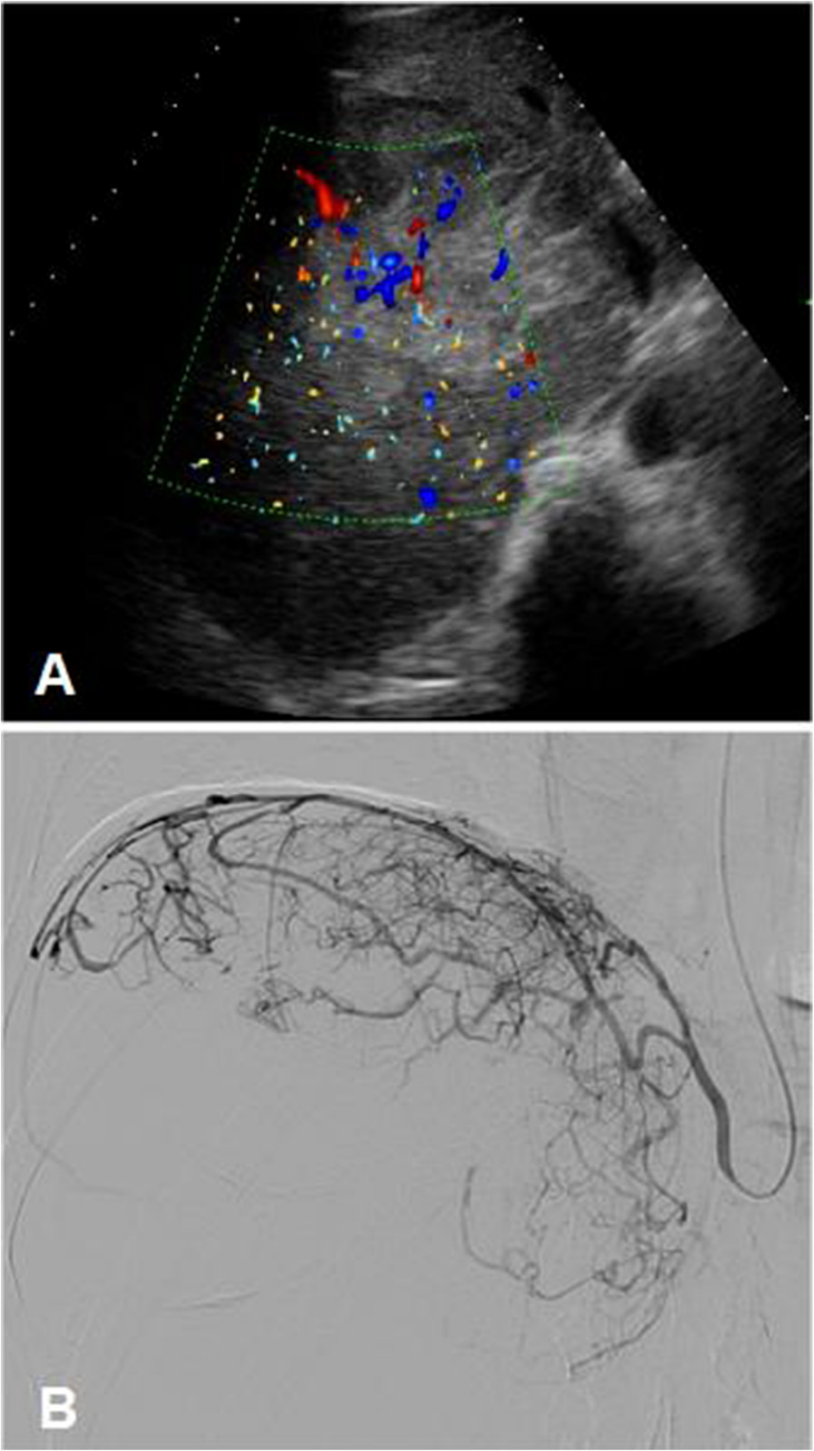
The large right lobe HCC was embolized via the right inferior phrenic artery. (A) Color Doppler sonography of the liver revealed a hypervascular HCC, which almost completely replaced the right lobe and extended to the diaphragm. (B) According to the initial angiogram the tumor was in part supplied by both the right inferior phrenic and the right hepatic arteries. Following selective catheterization and TAE the feeding arteries were closed off with gelatin sponge particles.
